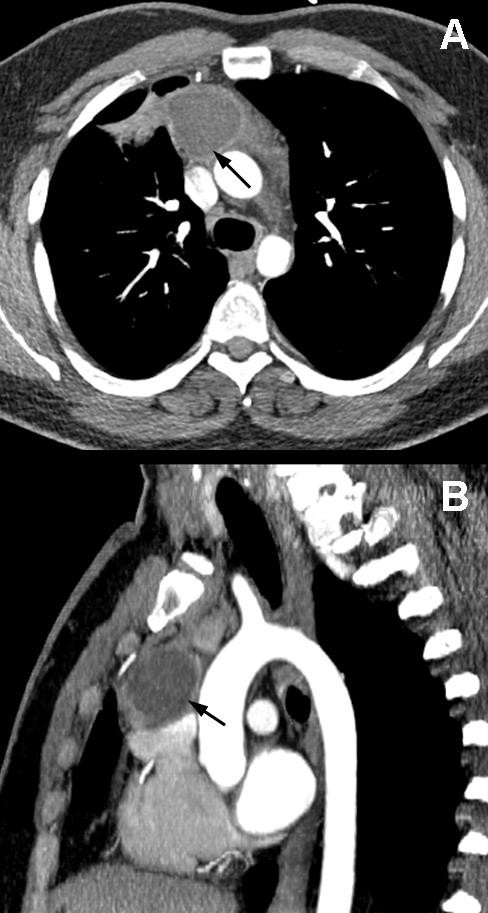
Chest CT -The arrows show the residual cystic mass detected between the right hilum and the ascending aorta connecting to the pericardium, the superior vena cava and the aorta on chest CT (A,B).
